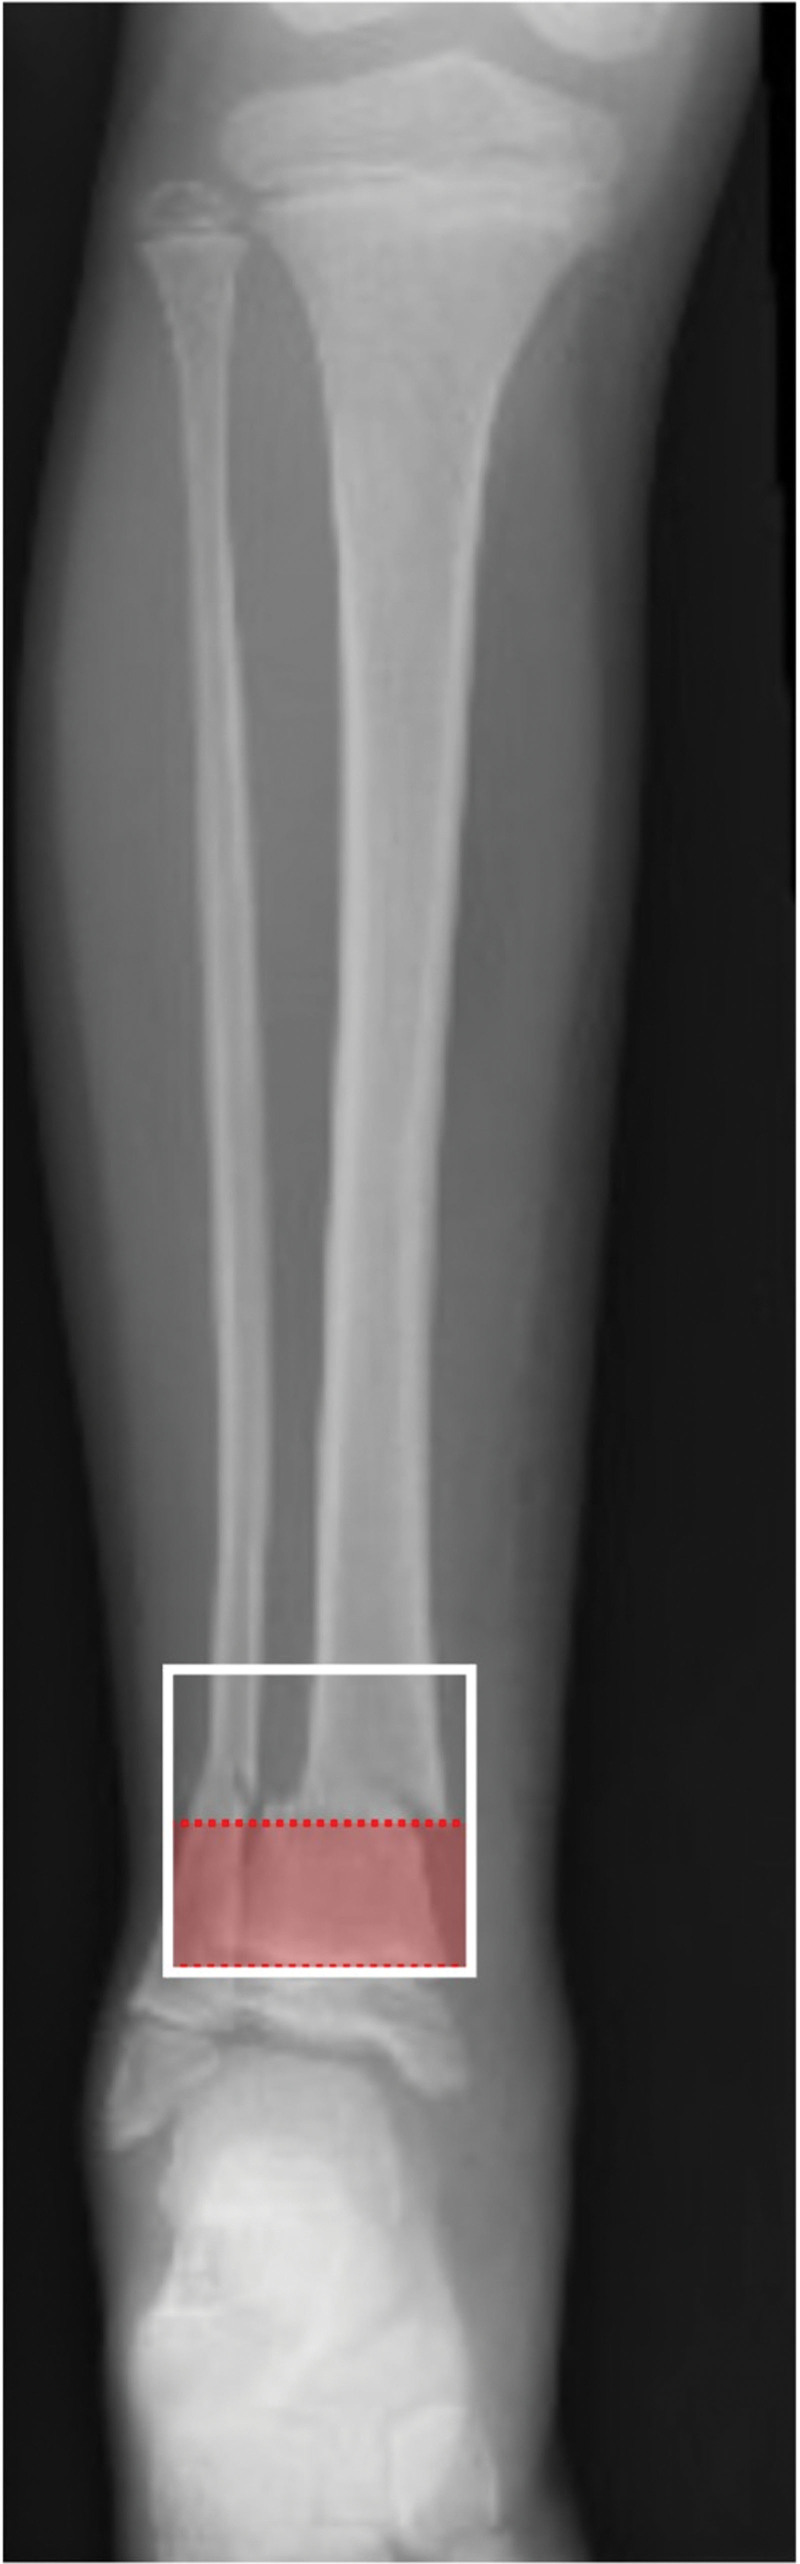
Radiograph showing fracture location area for short-segment diametaphyseal fractures. The white box is the epiphysis. The red shaded box, which is 50% of the width of epiphysis, is the defined location for short-segment diametaphyseal fractures.
